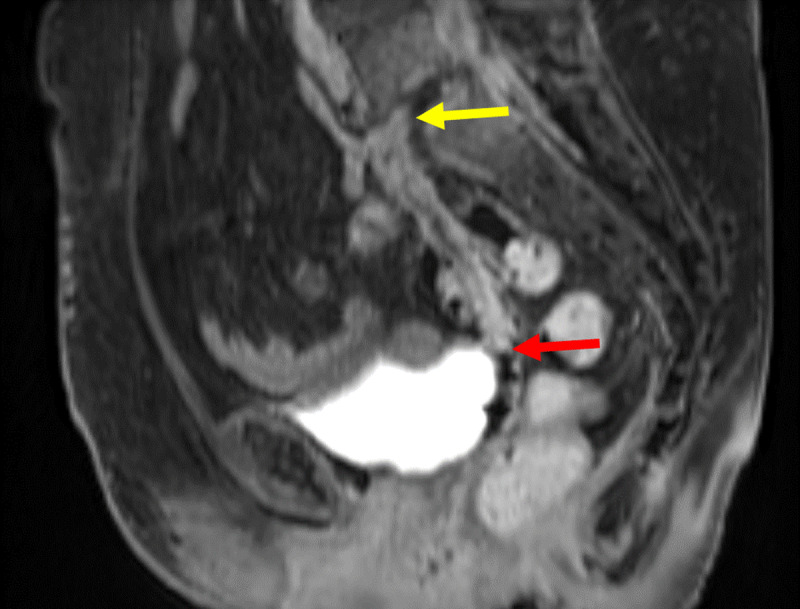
Sagittal contrast MRI in T1 fat saturation weighing: Full path of fistula from L5-S1 (yellow arrow) to the vagina. The fistula (red arrow) presents with a hyperintense wall surrounding a hypointense lumen.
